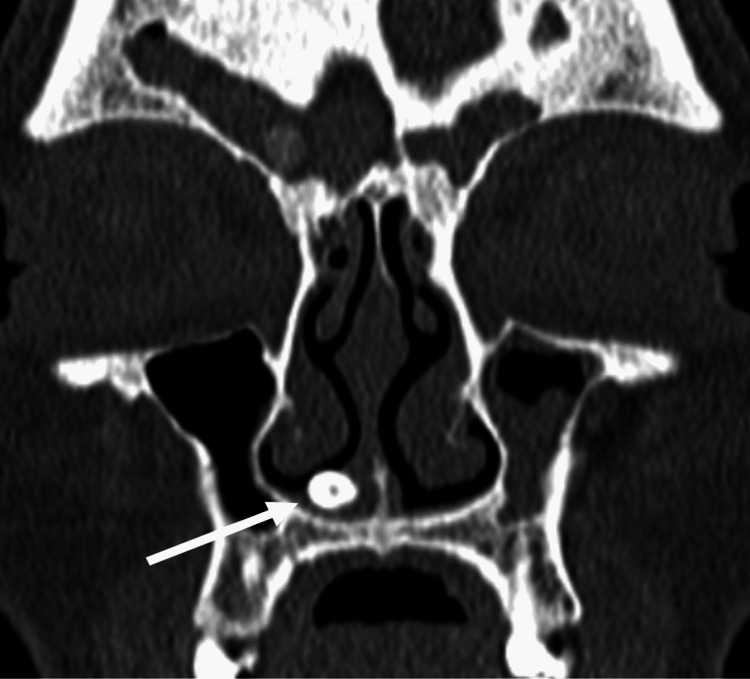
Preoperative coronal computed tomography imaging of the paranasal sinuses showing an intranasal tooth (white arrow) with a focal central radiolucency (resembling dental pulp) arising into the right nasal cavity.
